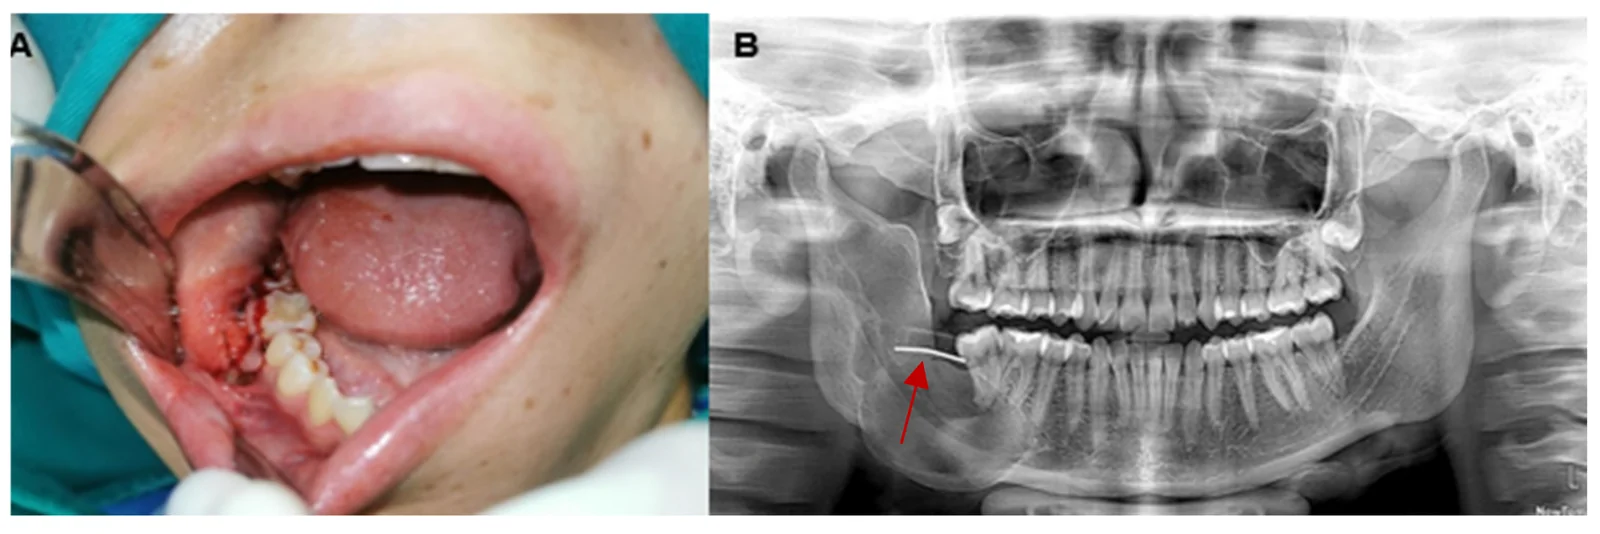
Placement of the decompression tube. (A) Intraoperative clinical photograph showing the tube secured within the surgical site. (B) Panoramic radiograph; the red arrow indicates the radiolucent shadow of the decompression tube at the site of the lesion.
